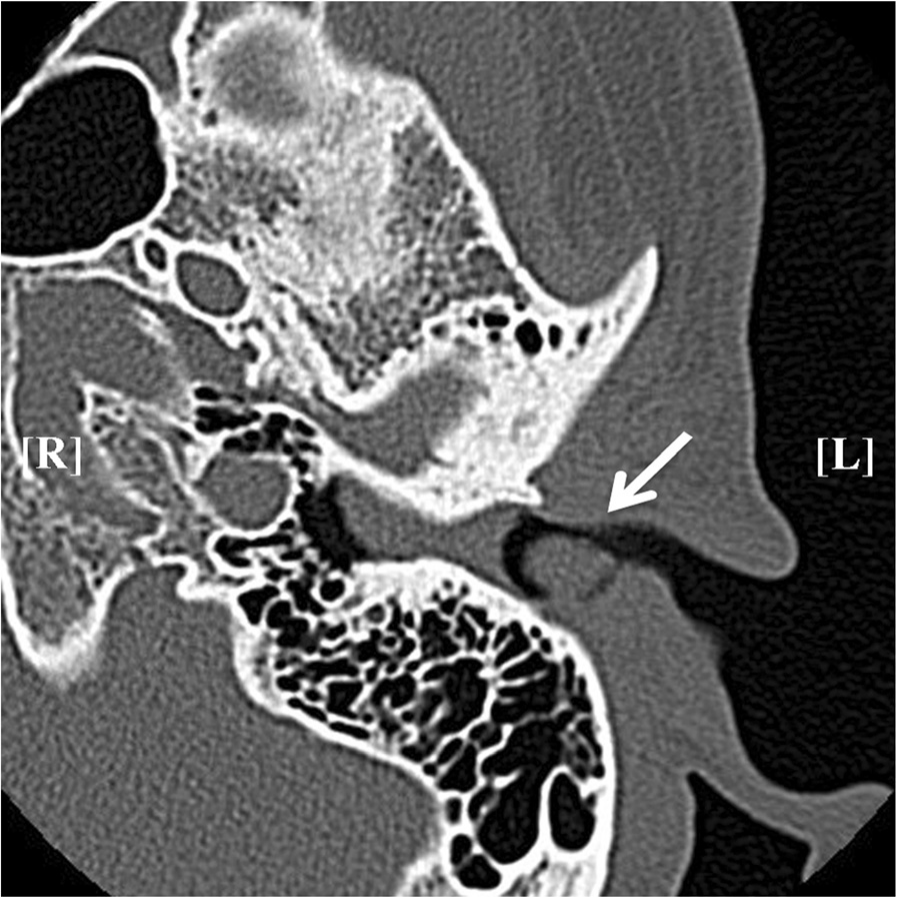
Computed tomography imaging: computed tomography showing the left ear canal mass (white arrow). The mass between the tumor and the tympanic membrane was thought to be earwax.
